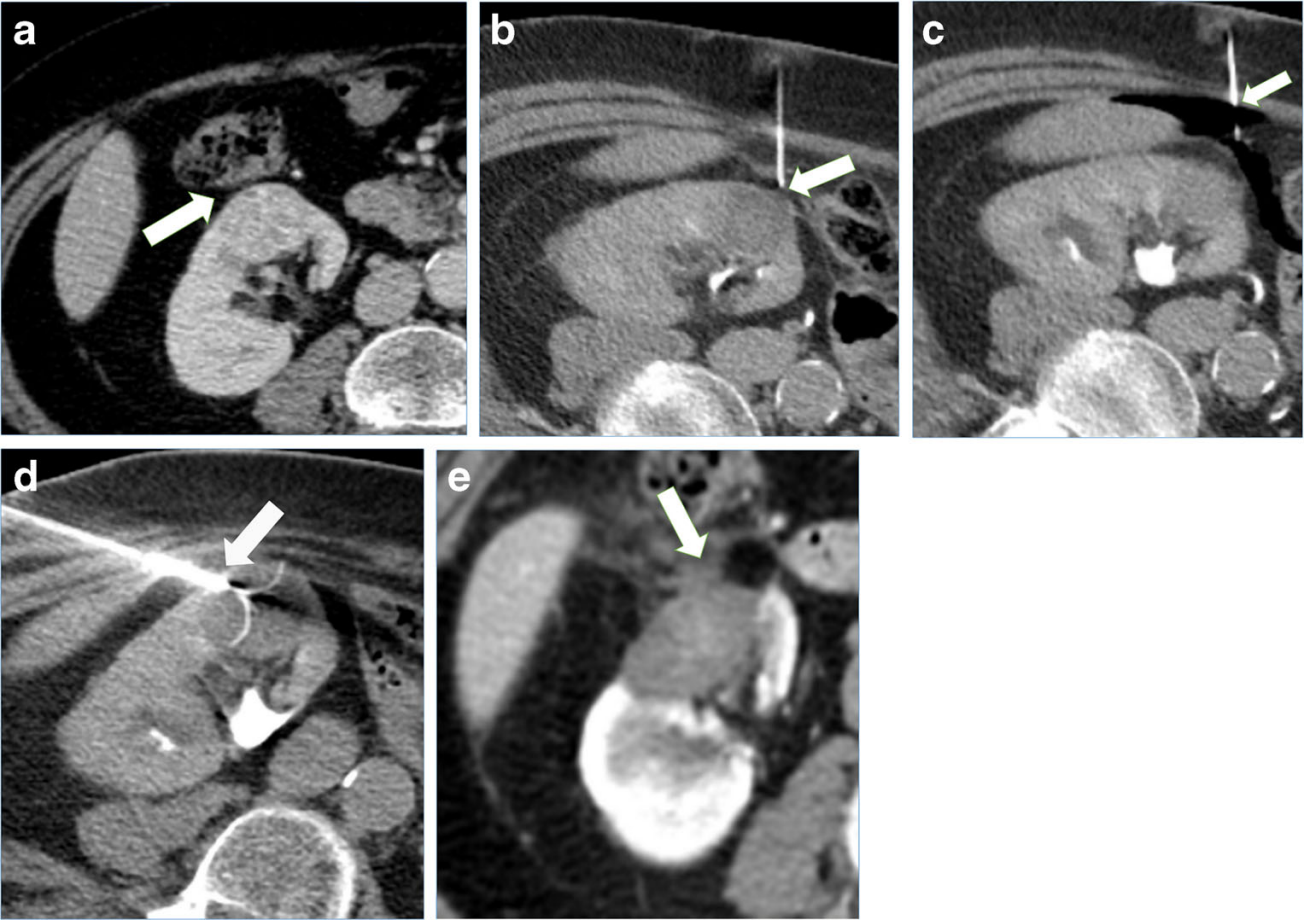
Treatment of a right renal tumour with image guided thermal ablation with the assistance of CO2 injection. a contrast enhanced CT demonstrating an anterior right renal tumour close to the colon (*arrow*). b patient was positioned in lateral decubitus and a small needle was inserted in between the tumour and the colon (*arrow*). c CO2 was injected through the small needle (*arrow*) in order to achieve colon displacement. d an umbrella-shaped radiofrequency electrode (*arrow*) was inserted into the tumour from the opposite site of the colon, and slight traction made in order to displace the organ. e a contrast enhanced CT performed 24 h after the ablation demonstrated good ablation of the tumour (*arrow*) without complications
